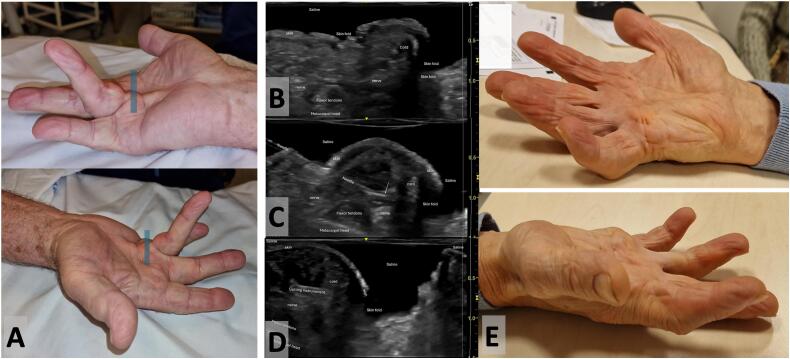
Dupuytren contracture in the case 2 affecting the ring finger. Fig. 2-A: preoperative condition. The blue rectangle represents the area that was covered by the probe to obtain the sonographic views of Fig. 2-B, 2-C and 2-D. Fig. 2-B: sonographic view under immersion showing on the same image small and large skin folds and bone, tendons, nerves and the Dupuytren cord. Fig. 2-C: targeted injection of local anesthesia around the cord. The white arrow represents the beveled needle tip. Fig. 2- D: sonography guided release of the cord. Fig. 2-E: clinical result, 12 days after the operation. Good recovery of finger extension, no skin rupture. (For interpretation of the references to colour in this figure legend, the reader is referred to the web version of this article.)
